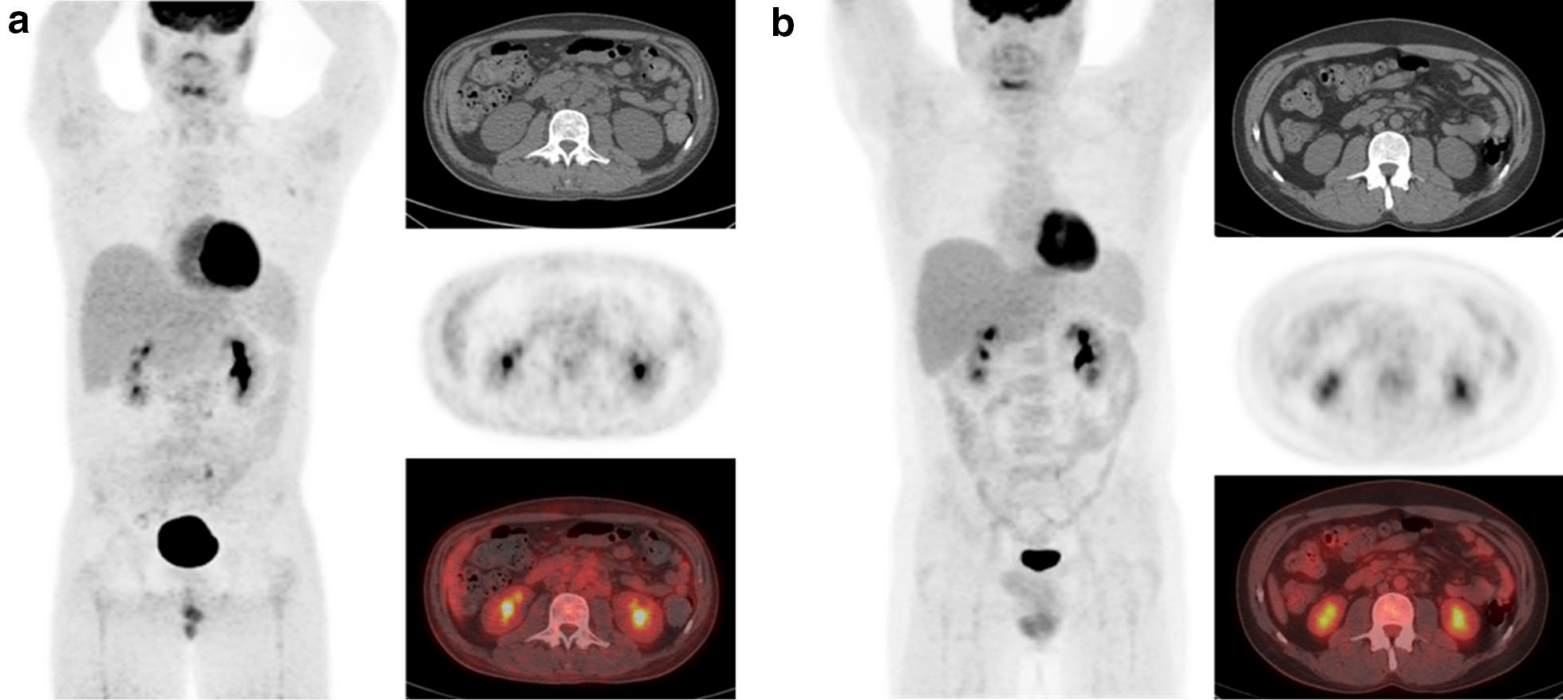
Radiology evaluation of disease burden after imatinib treatment. a PET-CT 4 weeks after initiation of imatinib treatment showing marked interval improvement in supradiaphragmatic and infradiaphragmatic lymphadenopathy as well as the skeletal/marrow disease burden. b PET-CT 6 months post imatinib treatment and 4 months reduced-intensity conditioning allogeneic stem cell transplantation shows no FDG-avid malignancy
